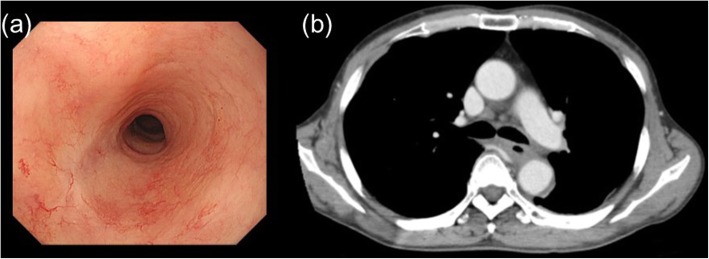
a Esophagogastroduodenoscopy revealed necrotic components and the disappearance of the esophageal tumor without erosion or ulceration of the esophageal mucosa at 13 months after initiating chemoradiotherapy; b computed tomography revealed that the airway deformation had resolved and the esophageal tumor was not observed at 13 months after initiating chemoradiotherapy
